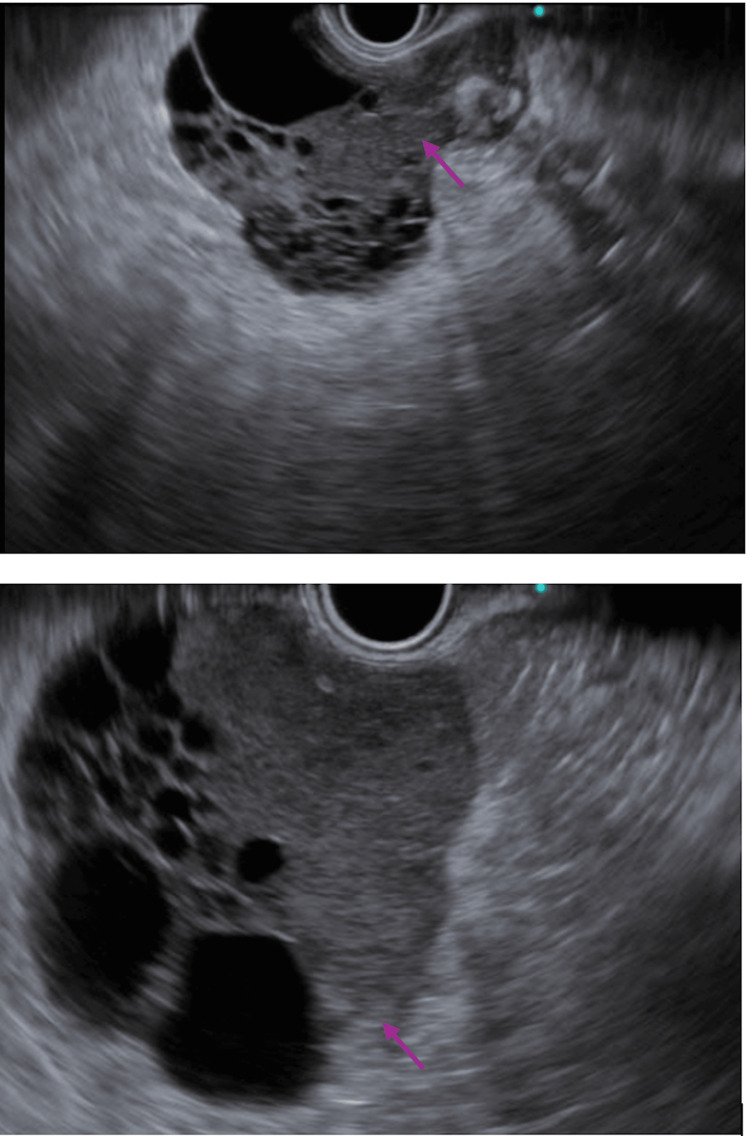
Endoscopic ultrasound demonstrated a 4×3 cm heterogeneous hypoechoic multilobulated lesion (arrows).
